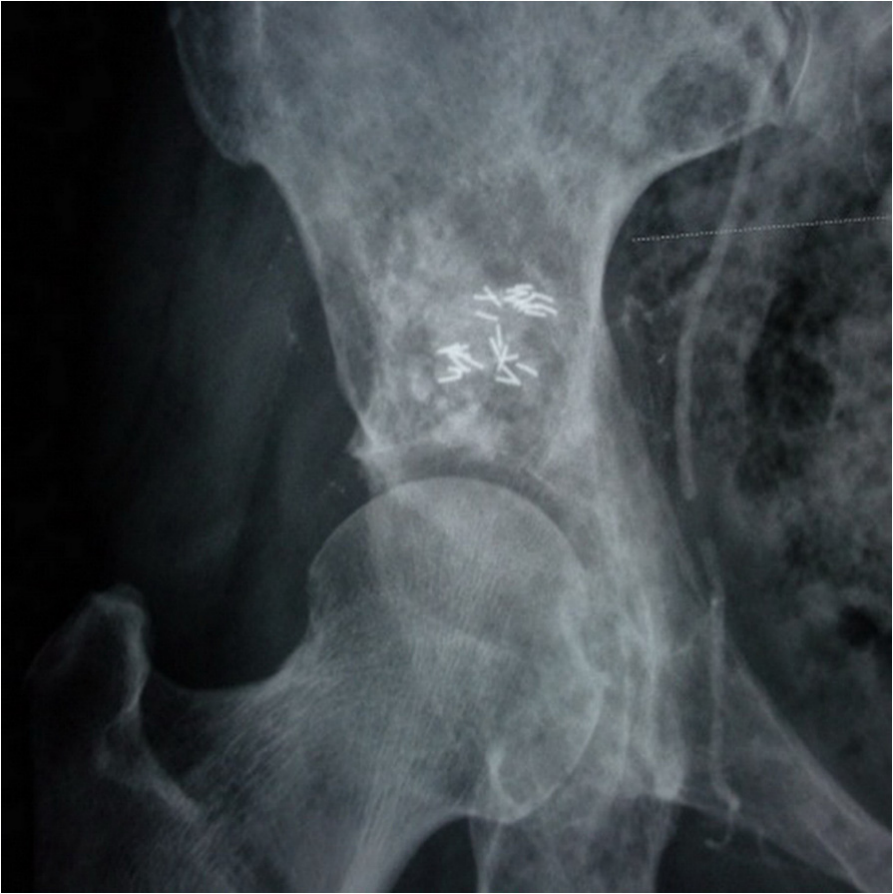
Postoperative radiographic review showing good ^125^I particle distribution and bone cement filling.
